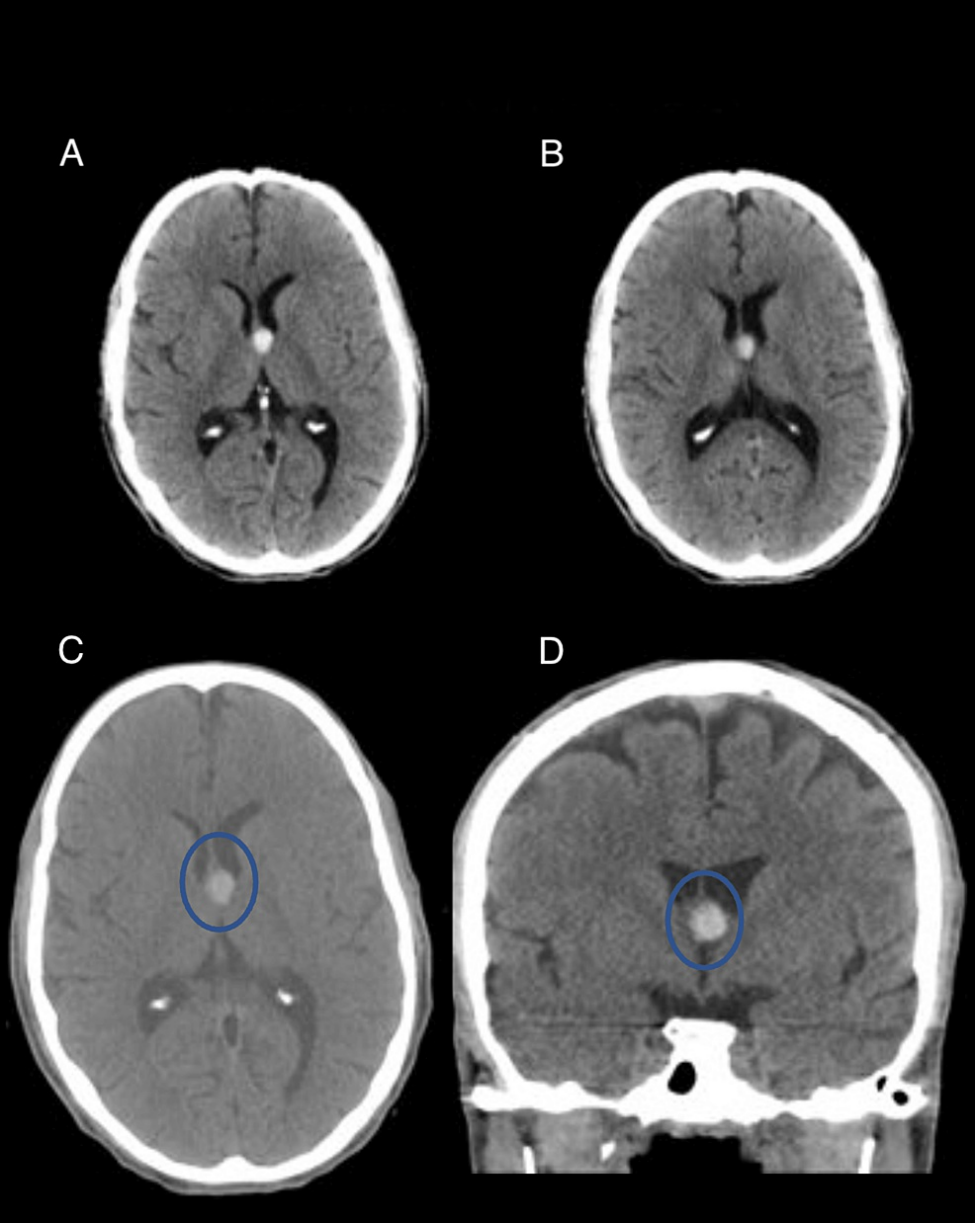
Four axial slices of a non-contrast head CT in a patient with a benign colloid cyst. Panels C and D highlight the benign protein-filled hyperdensity present in the Foramen of Monro (blue circles).
